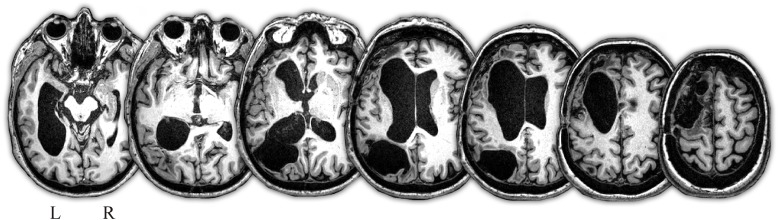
T1-weighted MRI axial images depicting extensive brain damage in the left hemisphere. There are two old cavitated lesions, one involving the left dorsolateral and medial frontal cortex with partial compromise of the anterior perisylvian language area (inferior frontal gyrus, pars triangularis), whereas the other lesion involved the left inferior parietal lobe (angular gyrus) and medial occipital lobe. There are also marked asymmetric dilatation of the lateral ventricle especially of the left frontal and temporal horns. Note that great part of the left perisylvian language core is spared by the lesions and no damage was observed in the right hemisphere. Images are shown in neurological convention. L, left; R, right.
